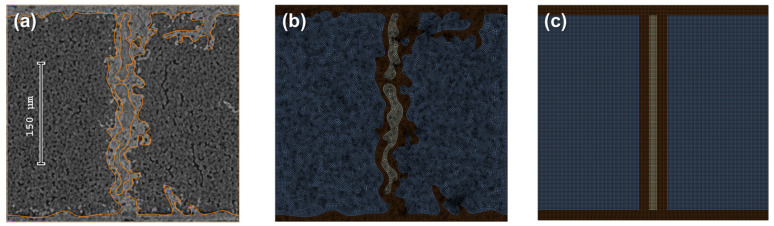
Intra-layer segment-RVEs. (a) CT image of a representative segmental section; (b) corresponding refined RVE; (c) simplified RVE.
